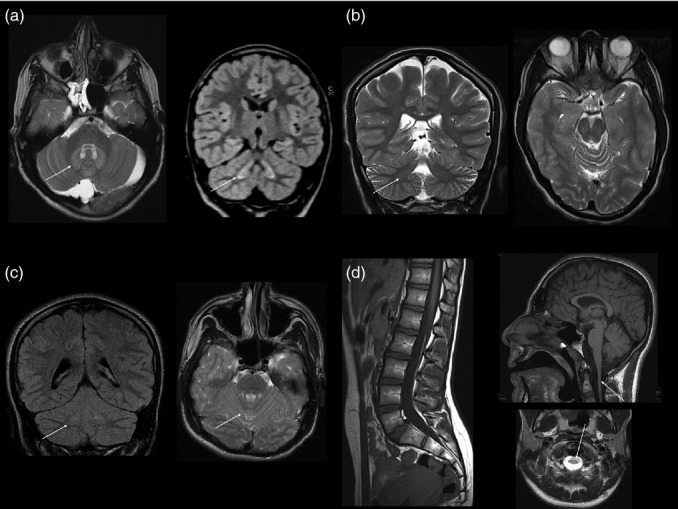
Other abnormalities on brain magnetic resonance imaging. (a) Axial T2-weighted and coronal volumetric fluid-attenuated inversion recovery images in patient 3 show bilateral symmetrical signal hyperintensity within the cerebellar deep nuclei and hilar white matter (arrow) and a retrocerebellar arachnoid cyst. This child also has a fatty filum terminale but normal position of the conus medullaris and no other spinal dysraphic features (not shown). (b) Coronal and axial T2-weighted images in patient 5-2 show prominence of the cerebellar hemisphere and vermian fissures. Note again the subtle symmetrical dentate nucleus changes (arrow). (c) Sagittal T1-weighted image (patient 1-1) shows normal position of the conus medullaris, a fatty filum terminale, and normal spinal cord volume. (d) Sagittal T1- and axial T2-weighted brain images (patient 2) show global lack of cerebral volume with slightly thin corpus callosum and brainstem and spinal cord hypoplasia.
